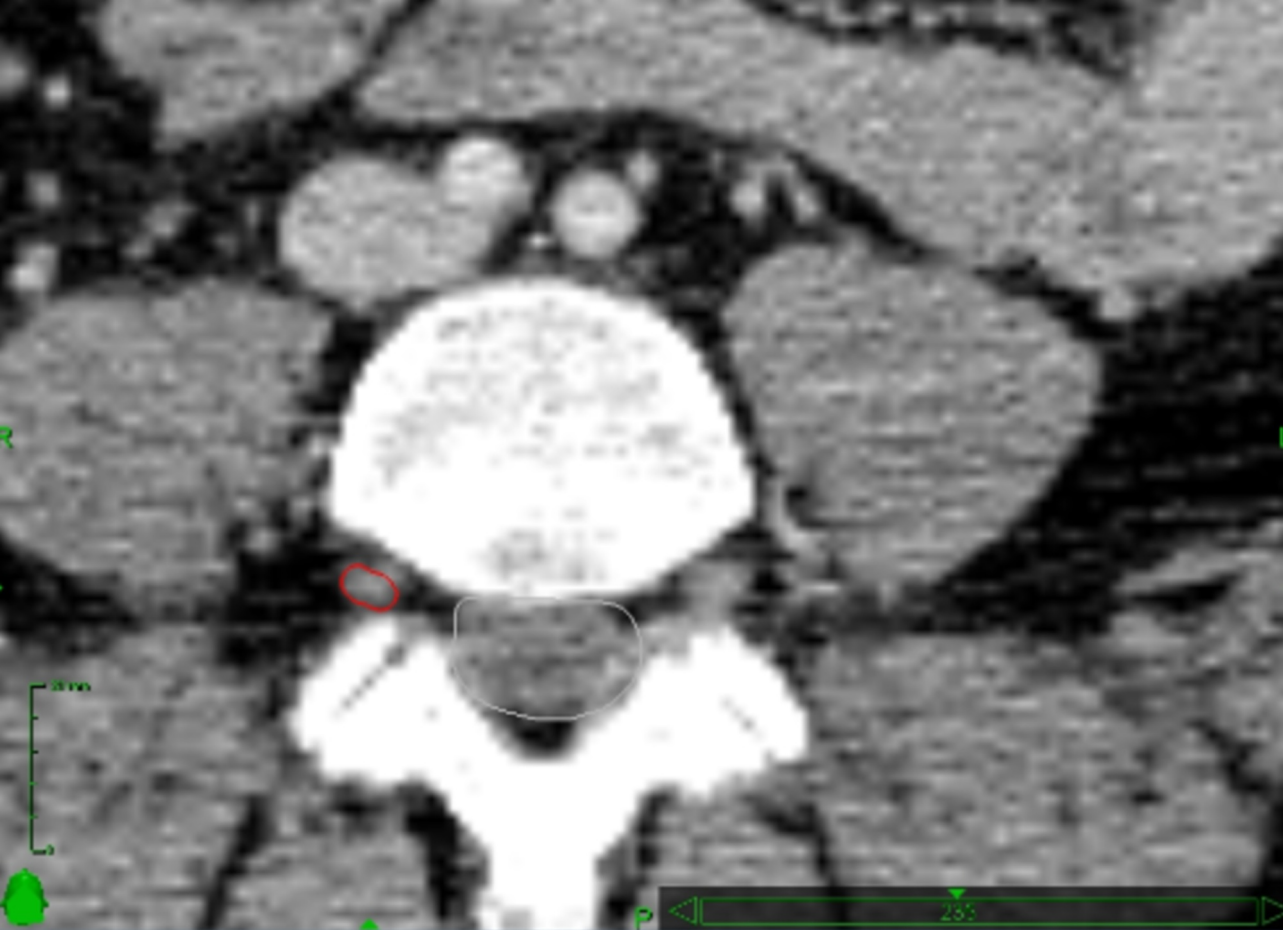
Target identification in the CT scan used for treatment planning The dorsolateral segment of the right L4 nerve root is contained within the red line. The target is located inside the L4 foramen.
